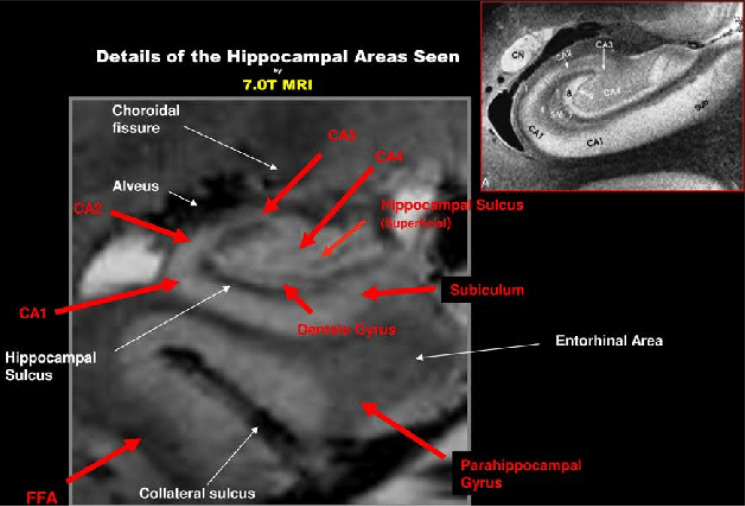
Details of hippocampal areas are clearly visible on 7T MRI. Most of the sub-components of the hippocampal proper near the head of the hippocampus, CA1, CA2 etc. can be clearly seen together with the surrounding parahippocampal cortices such as the subiculum, part of the entorhinal cortex, as well as the fusiform area (FFA).
